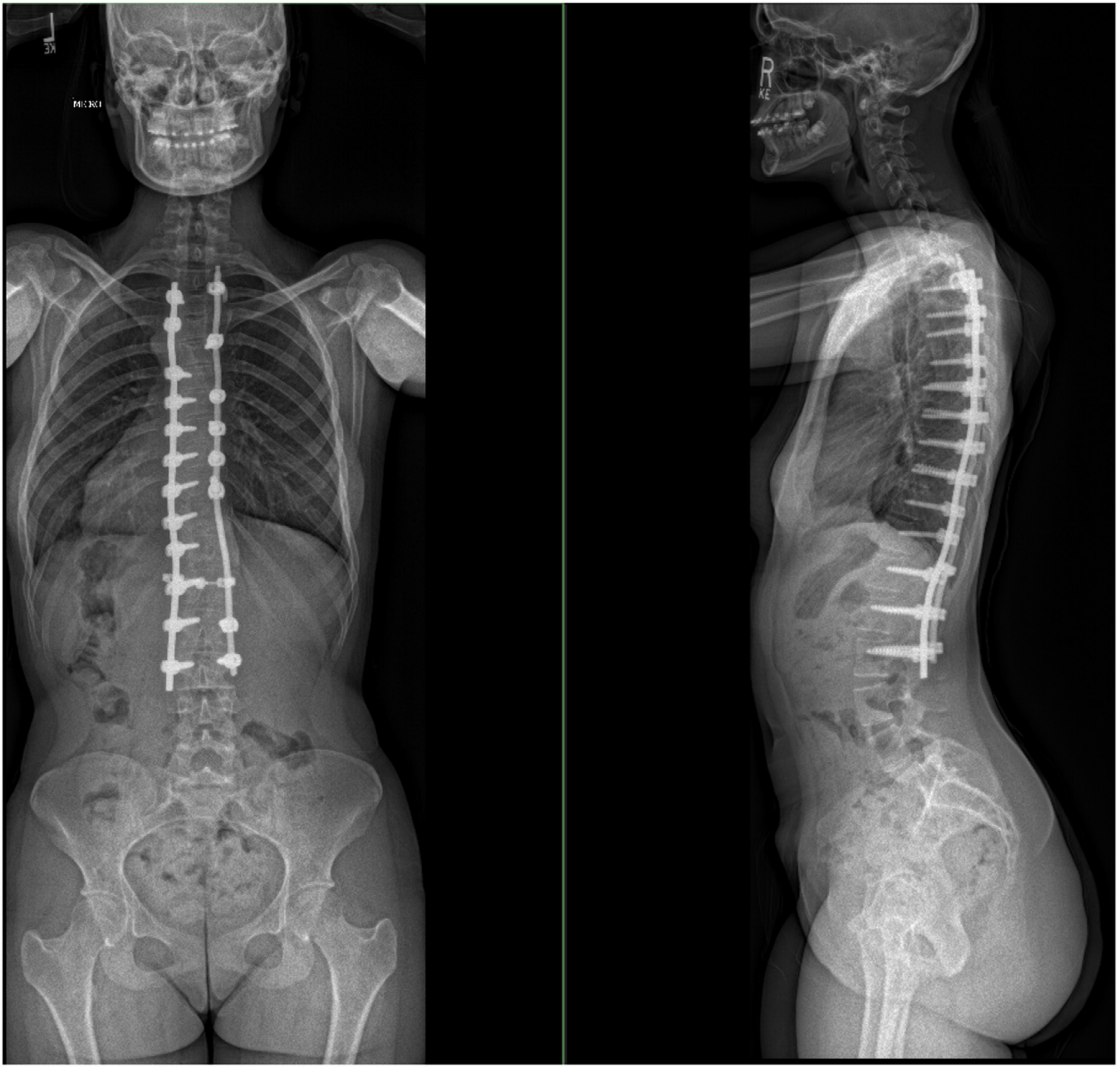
Anteroposterior and lateral plain radiograph showing correction of scoliosis with a posterior instrumentation from T3 t– L3.
